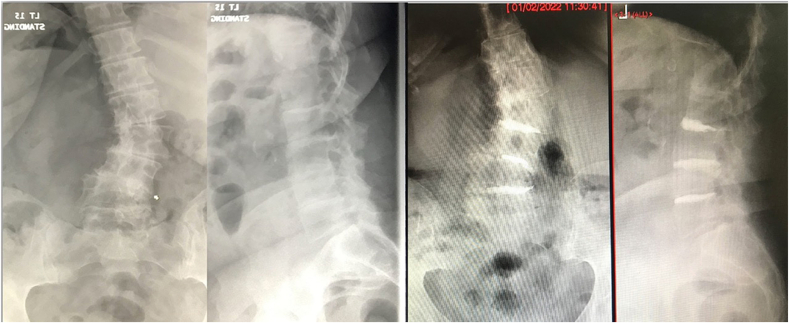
78 year old lady with degenerative scoliosis, back pain 5/10 and leg pain (R L34 dermatome) 9/10. Had L34 extra-foraminal decompression and open CD, percutaneous CD at L23 and L45. No peri-operative or early complications. A & B: Pre-operative radiographs. C & D: Two-year post-operative radiographs with restoration of disc height at affected levels, stabilisation of the scoliosis. VAS was 3/10 and ODI was 22%.
